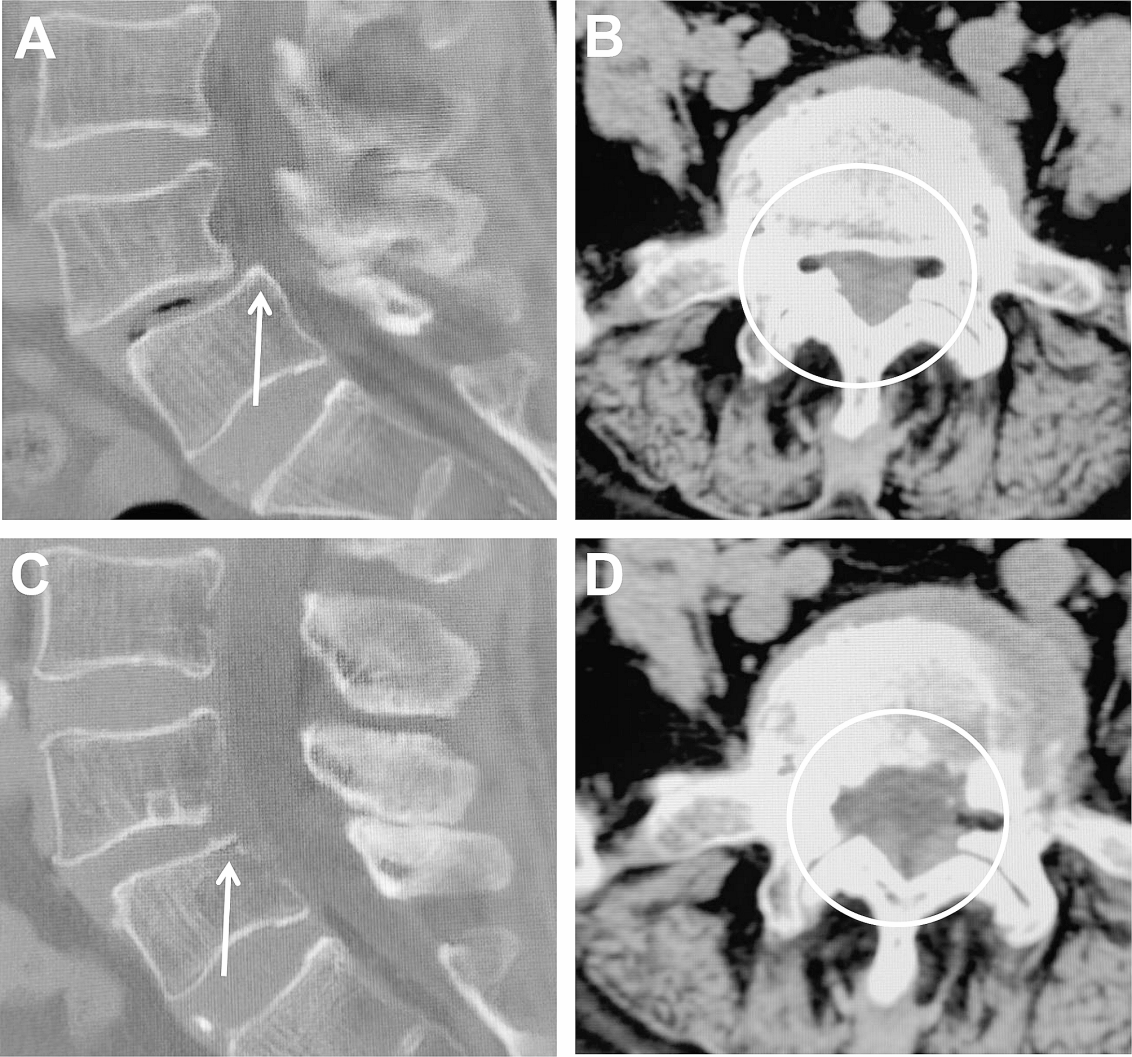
Pre- and postoperative CT. (A, B) Preoperative CT showed that the PRSVB (white arrow), the central spinal canal stenosis, foraminal stenosis and lateral recess stenosis (white circle). (C, D) On the third day after operation, the CT showed complete decompression of the nerves and removal of PRSVB (white arrow). The ventral and dorsal edges of the spinal canal became smooth and continuous. The lateral recess, foramen, and central spinal canal were enlarged (white circle)
